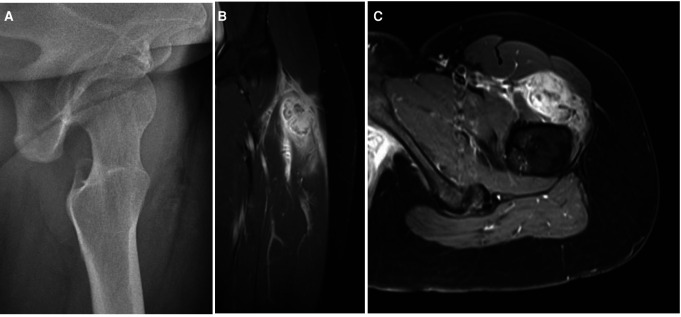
(A) Radiograph and (B,C) MRI finding in an adolescent with a circumscribed myositis ossificans lesion on the left thigh, one month after the triggering event.
